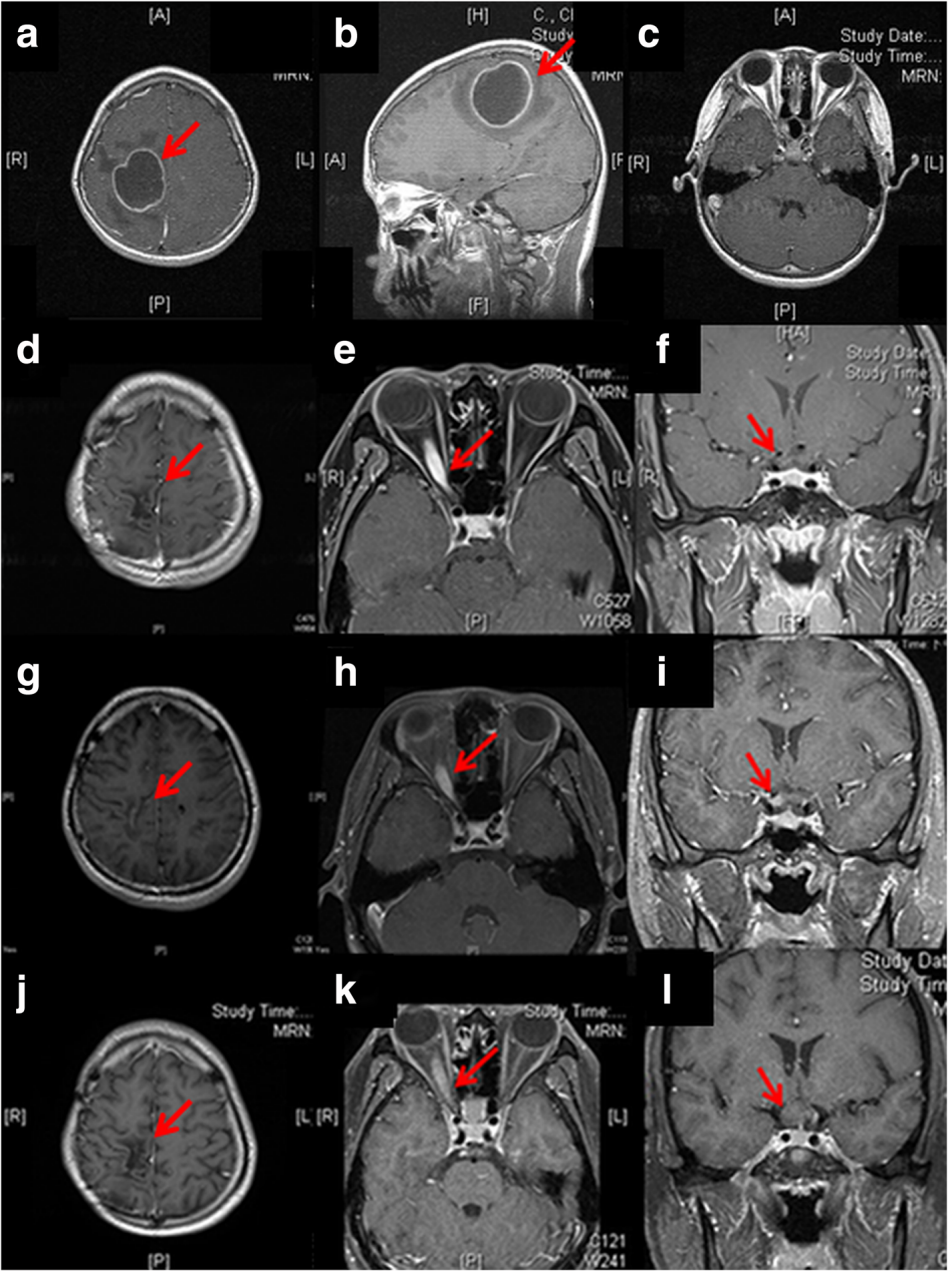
T1 weighted contrast – enhanced MRI showed (a, b) original right frontoparietal glioblastoma (c) without right optic nerve involvement. At the initial symptom onset, T1 weighted contrast – enhanced MRI with fat suppression showed (d) no evidence of brain tumor recurrence but (e, f) right pre-chaismatic enhanced fusiform optic nerve tumor. At 3 months after symptom onset, T1 weighted contrast – enhanced MRI with fat suppression showed (h, i) right fusiform enlargement and an enhanced optic nerve tumor intraorbitally extending towards the optic chiasm even (g) no brain tumor recurrence. At 11 months after symptom onset, T1 weighted contrast – enhanced MRI with fat suppression showed (k, l) right optic nerve tumor extending to the optic chiasm and progressing in size (j) without brain tumor recurrence
